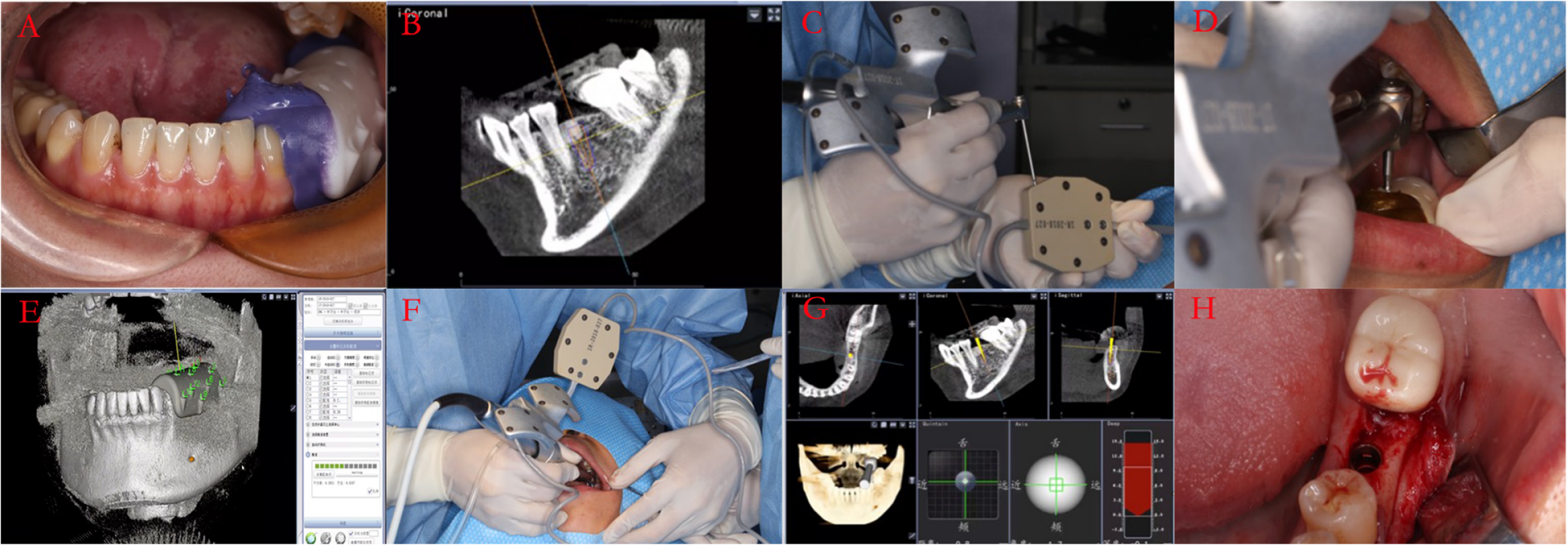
The process of dynamic navigation. a The registration device was fixed onto the operating arch with a silicone elastomer when taking the CBCT scanning. b The optimal 3D implant position was planned in the dental implant navigation system software. c Matching the reference device. d Matching the registration plate with the handpiece. e The view of matching the registration plate in the software. f The process of drilling under navigation. g The view of real-time tracking under navigation in the software. h Oral image of implant placement
